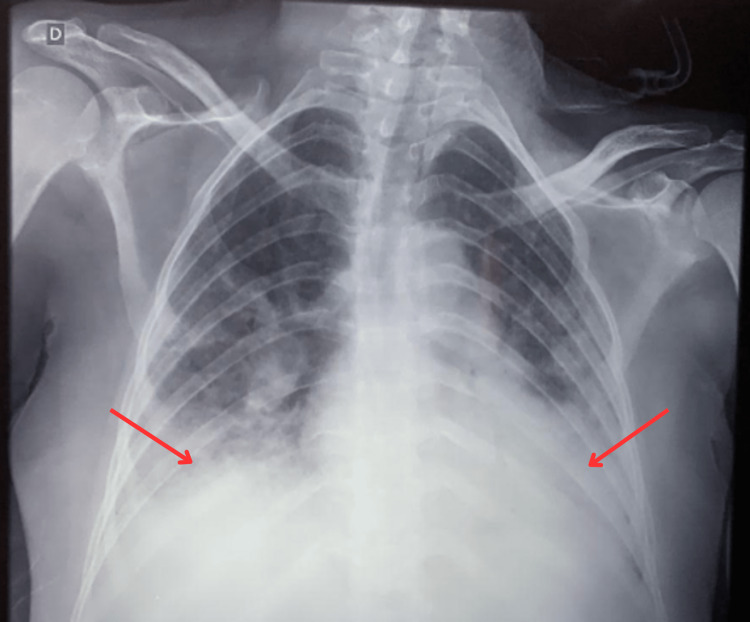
Initial chest radiography registered in the anteroposterior view showing basal consolidative opacities in both lungs, as indicated by the red arrows.
